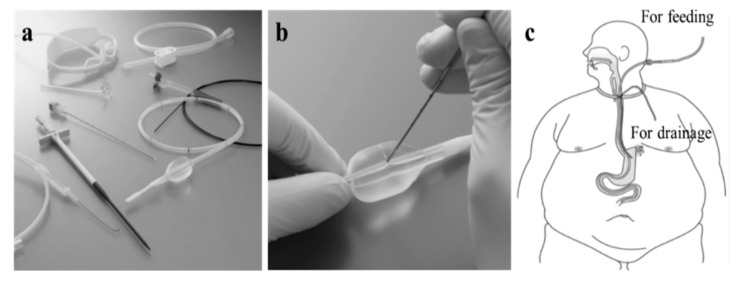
(a) PTEG kit (SB-KAWASUMI LABORATORIES, INC., Akita, Japan). (b) The rupture-free balloon is not ruptured by needle puncture. (c) Image of the double percutaneous transesophageal gastro-tubing.
